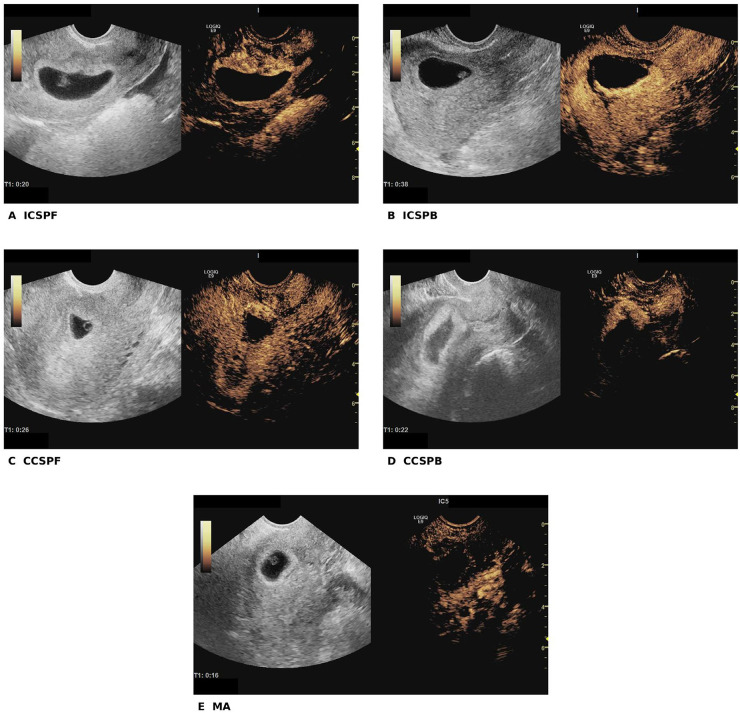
Representative CEUS images of the five enhancement-pattern subtypes in cesarean scar pregnancy. (A) ICSPF; (B) ICSPB; (C) CCSPF; (D) CCSPB; and (E) MA. In each panel, the grayscale transvaginal ultrasound image is shown on the left and the corresponding contrast-enhanced ultrasound image is shown on the right.
